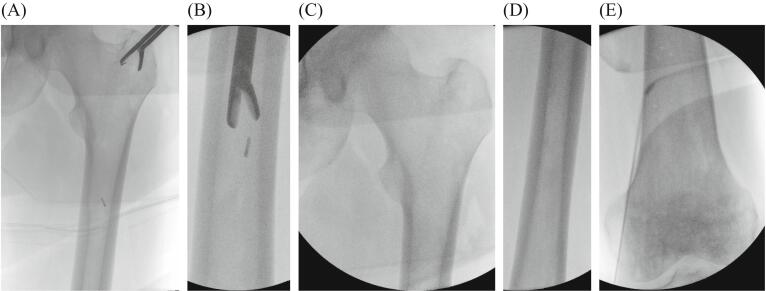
Intraoperative radiographs during the revision surgery, showing fluoroscopic identification (A) and removal of metal debris parts (B) without any residues at the proximal femur (C), shaft (D) or condylar region (E).
